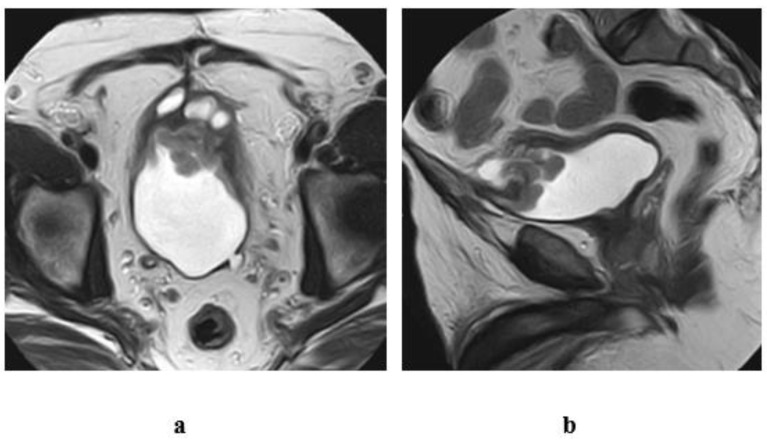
A case of urachal adenocarcinoma (enteric type) in a 60-year-old male patient, complaining of hematuria. T2 w MRI of the pelvis (a) axial, (b) sagittal views: inhomogenous midline mass of mixed cystic and soft tissue components, infiltrating the dome of the bladder and connected superiorly to the umbilicus with a track displaying hypointense SI, representing the fibrous remnants of urachus.
